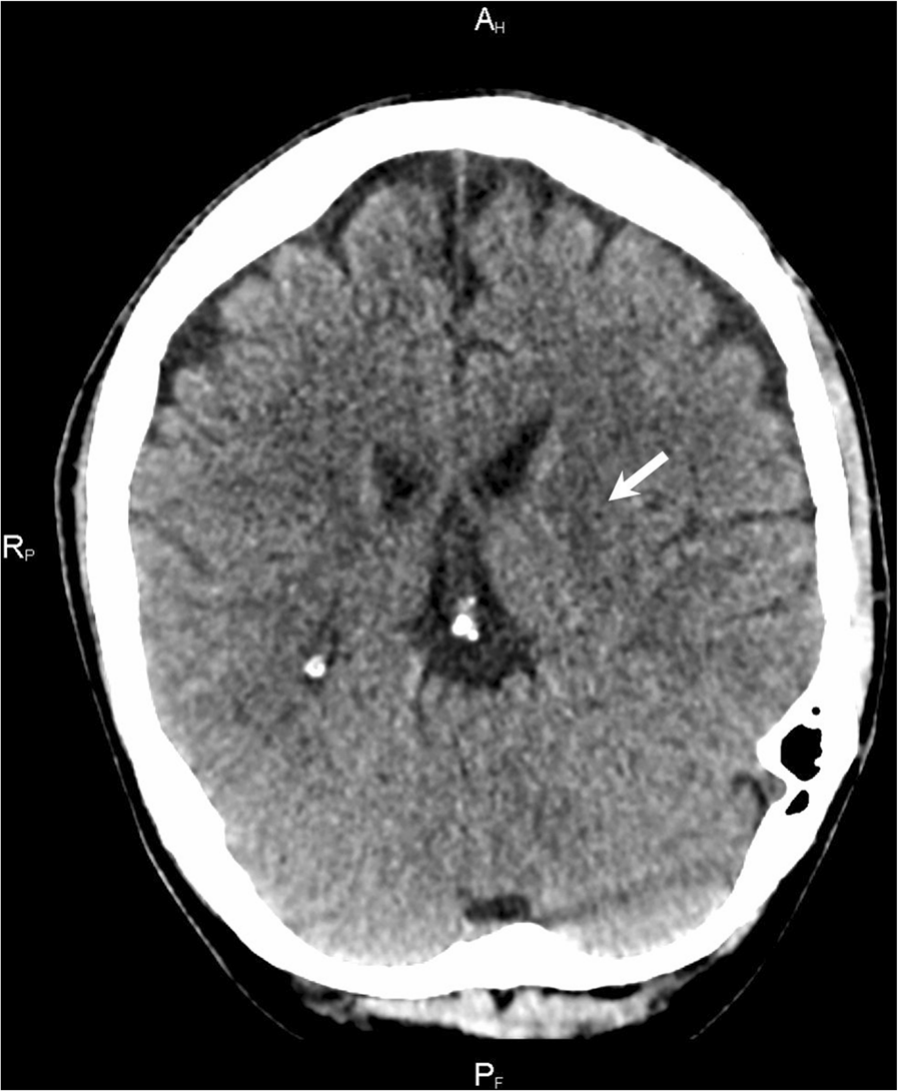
Native computed tomography scan of the brain. Slight hypodensity in the basal ganglia mainly on the left side (arrow) can be seen (post-ischemic changes after prolonged hypoxemia).
